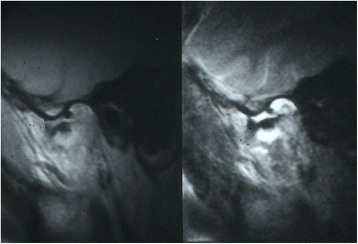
Sagittal proton density-weighted image shows a condylar head fracture. It also demonstrates that the disc is deformed and anteriorly displaced. Sagittal T2-weighted image demonstrates a disruption of the oblique line representing capsule. It is thought to be a capsular tear. Also, joint effusion and hemarthrosis are found in the superior and inferior joint space
